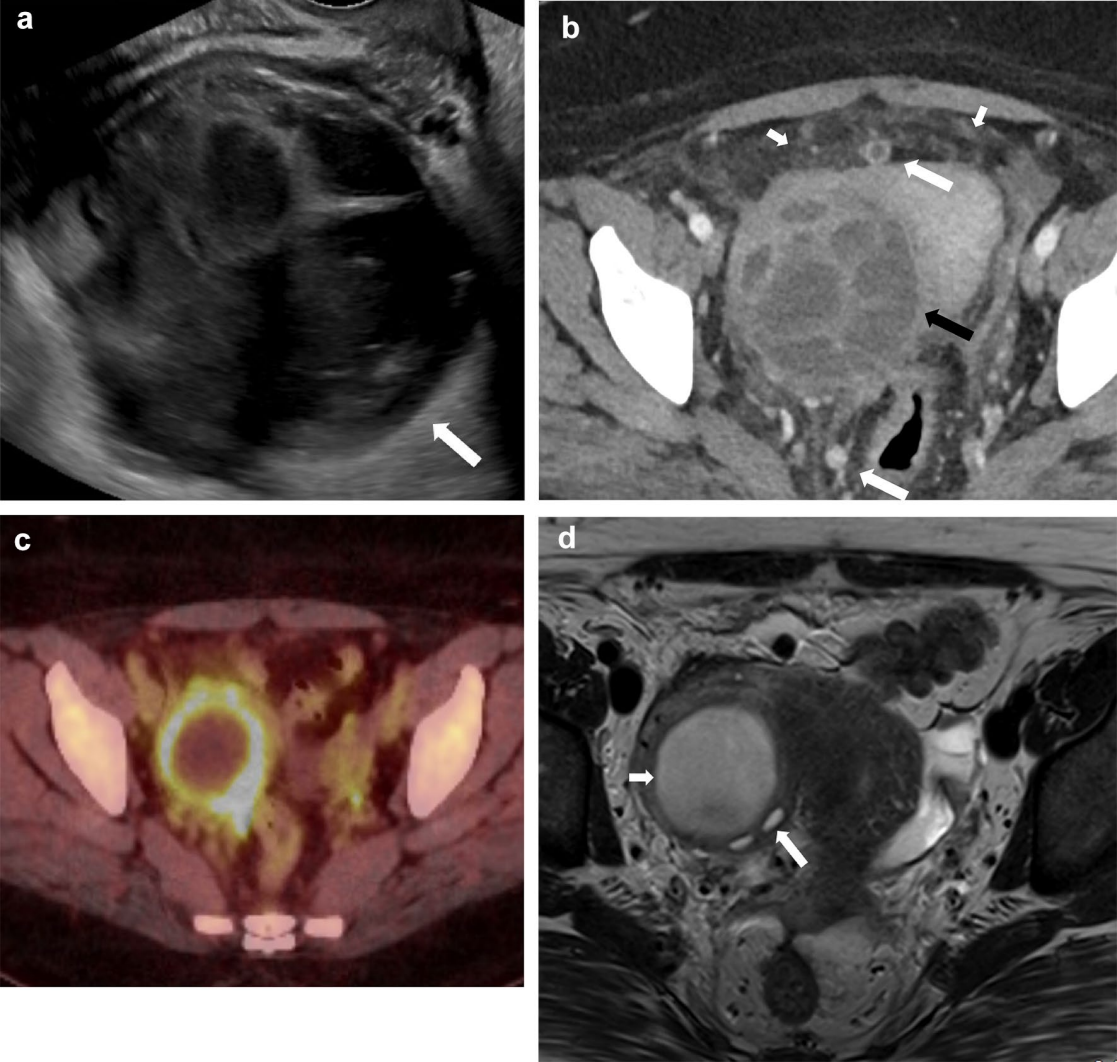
A 46-year-old female with dropped gallstones and a resultant pelvic abscess presenting as a pelvic mass. a Ultrasound of the pelvis showed a complex right adnexal mass (arrow). The right ovary was not visualized separately. CT abdomen pelvis was performed. b Axial CECT image showing a complex multiseptated right adnexal mass demonstrating loss of fat planes with the uterus (black arrow), associated fat stranding (short white arrows), and rounded peritoneal nodules (long white arrows). The patient was treated with antibiotics and underwent laparoscopy, but the procedure was aborted as the surgeon saw multiple peritoneal nodules in the pelvis. c) PET/CT was performed post laparoscopy, Axial PET/CT image shows uptake in the periphery of the mass (arrow). MRI of the abdomen was performed 2 weeks after the CT study. d) Axial T2 weighted MRI image showing interval decrease in loculations in the cyst (short arrow). The cyst is inseparable from the right ovary (long arrow). The patient underwent a hysterectomy, bilateral salpingectomy, and right oophorectomy. On pathology, the multiple peritoneal nodules seen on imaging were consistent with gallstones. The right adnexal mass showed inflammatory contents and golden-brown sediment within it, consistent with bile
